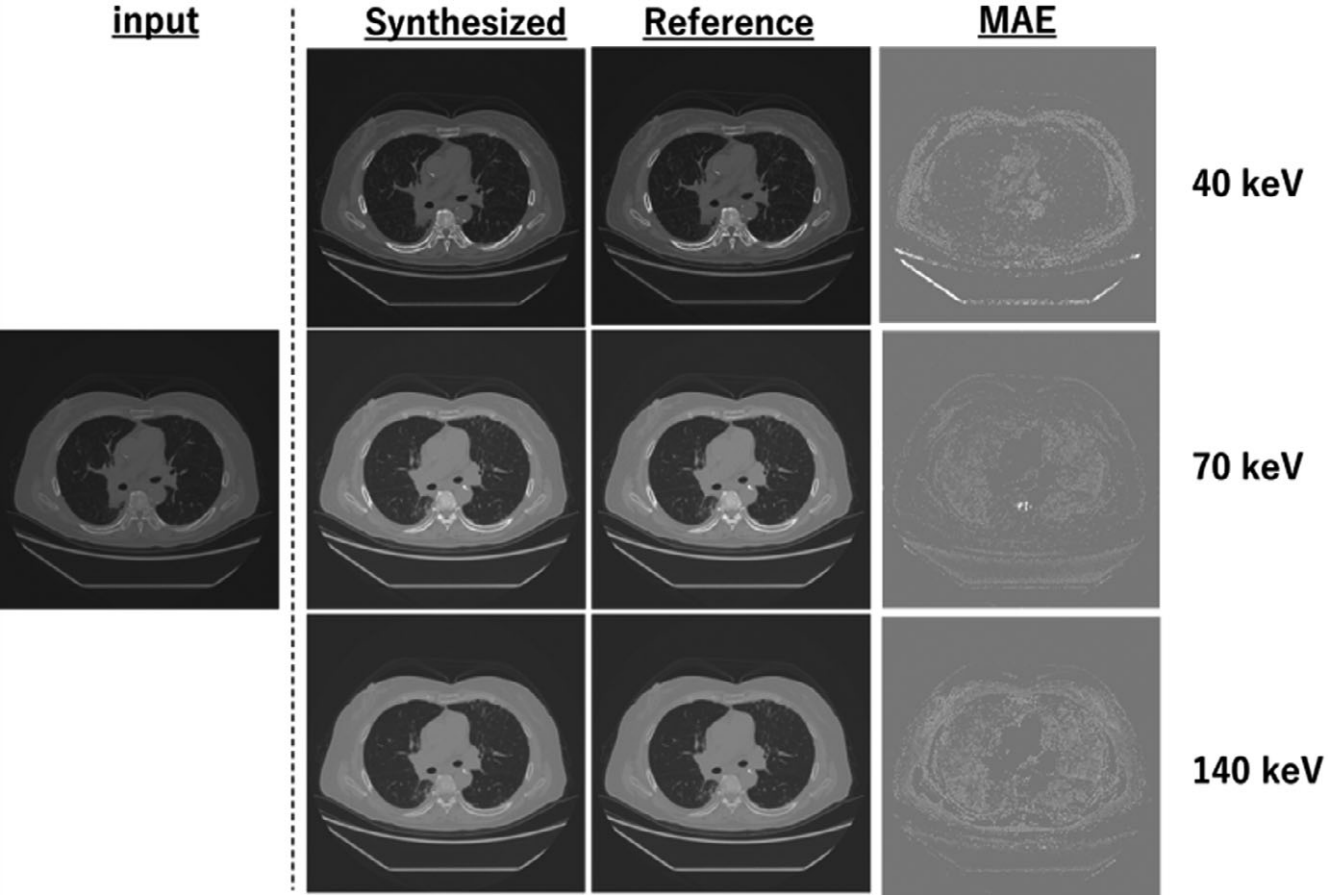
Samples obtained from monoenergetic CT image generation at the chest level: input image is the equivalent kV‐CT image, synthetic and reference images are the monoenergetic CT images at 40, 70, and 140 keV, and MAE is the difference between the synthesized and reference monoenergetic CT images.
